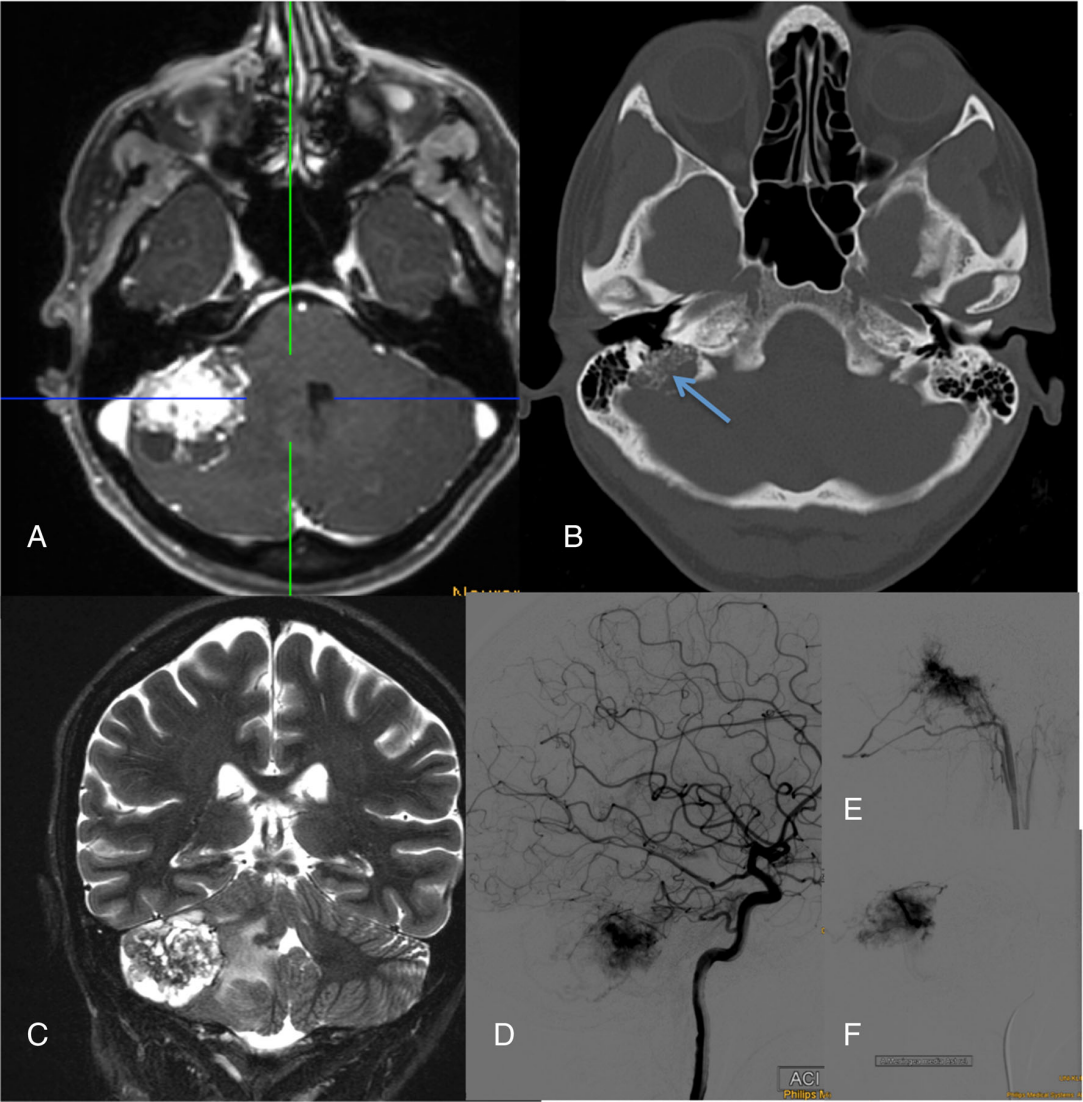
Endolymphatic sac tumor (ELST) in a typical location with strong enhancement (a), calcific spiculae on CT (b: arrow), inhomogeneous signal due to calcifications and cysts on T2-weighted images (c), and intense vascular blush with supply via branches of the ICA (d), ascending pharyngeal artery (e), and middle meningeal artery (f)
